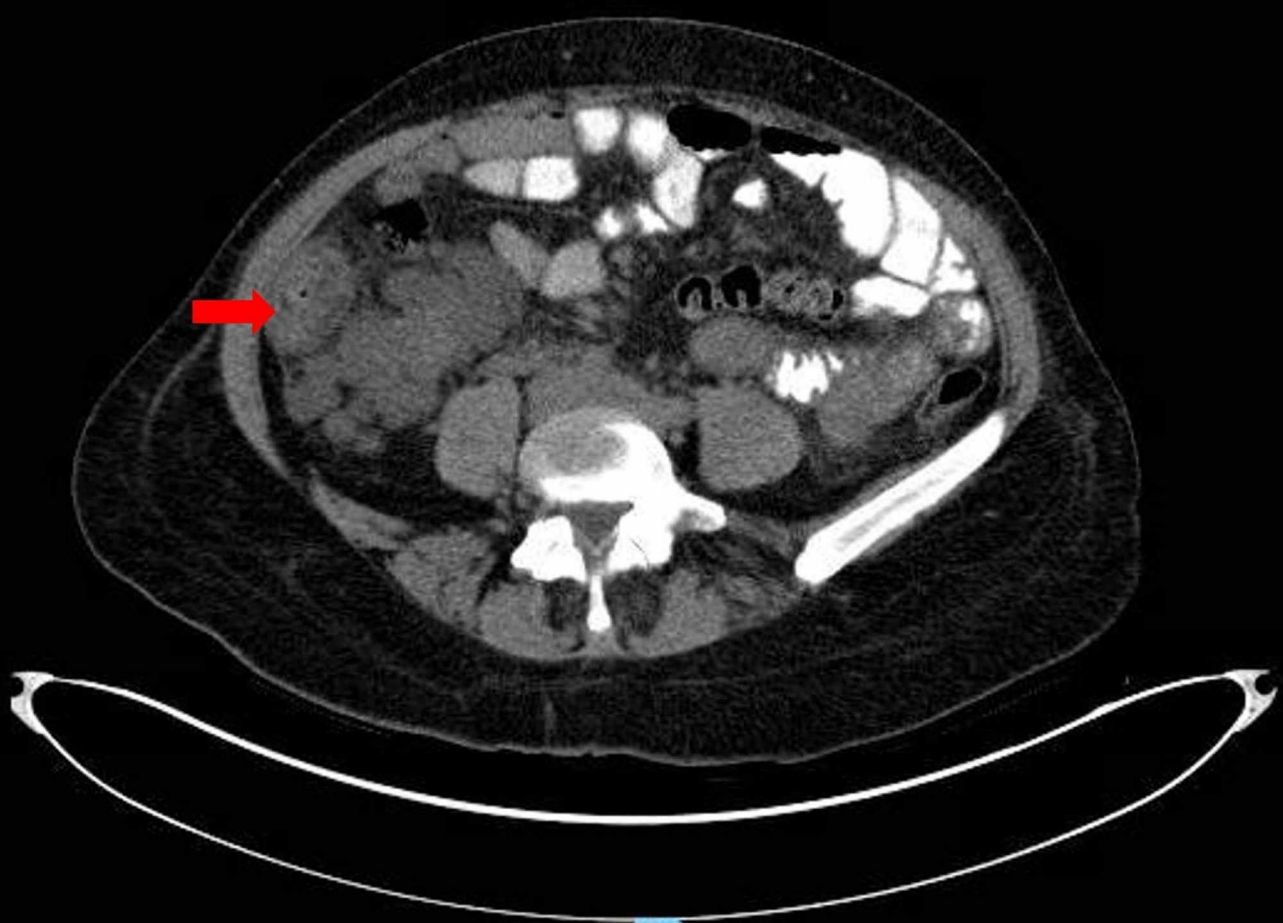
Computed tomography (CT) scan of abdomen and pelvis showing thickening of wall of ascending colon with mild inflammatory changes (red arrow), suggestive of colitis of the ascending colon
